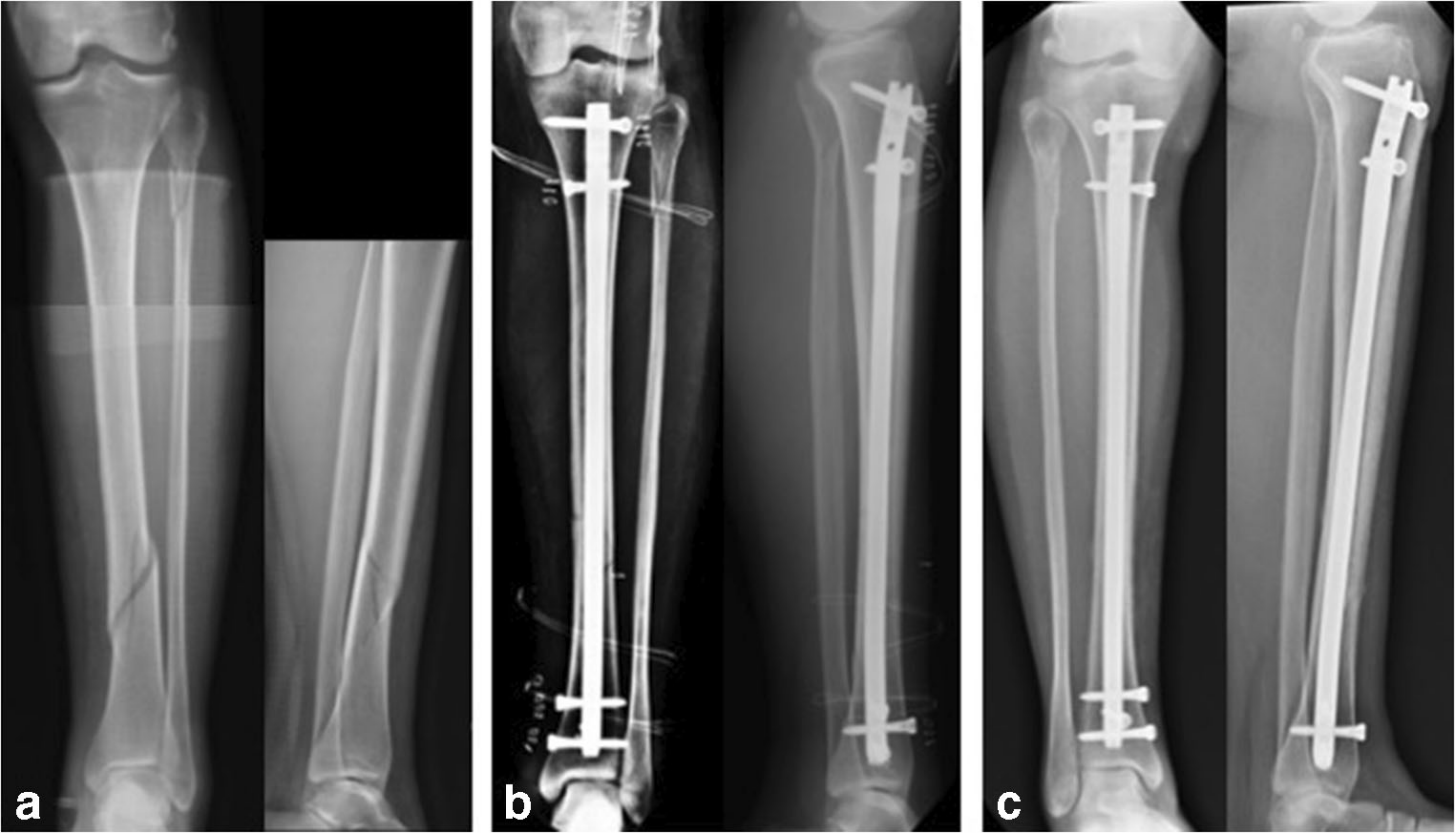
Patient 3: a Preoperative x-rays of distal third tibial diaphyseal fracture. b Post-operative x-rays with an intramedullary nail. c 7-month follow-up x-rays showing distal tibial bone healing. The score calculated was 1 point (see Table 9)
